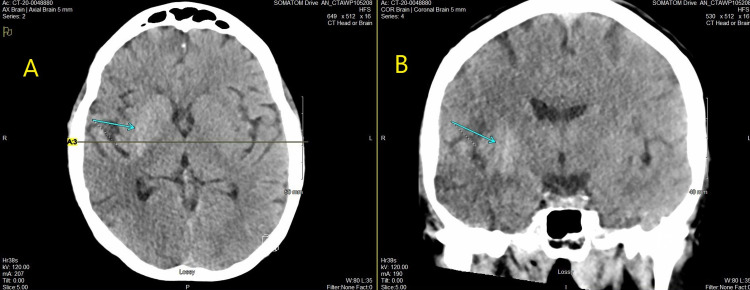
CT non-contrast scan demonstrating abnormal attenuation. (A) axial view with arrow indicating attenuation. (B) coronal view with arrow indicating attenuation. (A) Axial computed tomography (CT) non-contrast scan indicating abnormal attenuation. (B) Coronal computed tomography (CT) non-contrast scan indicating abnormal attenuation.
